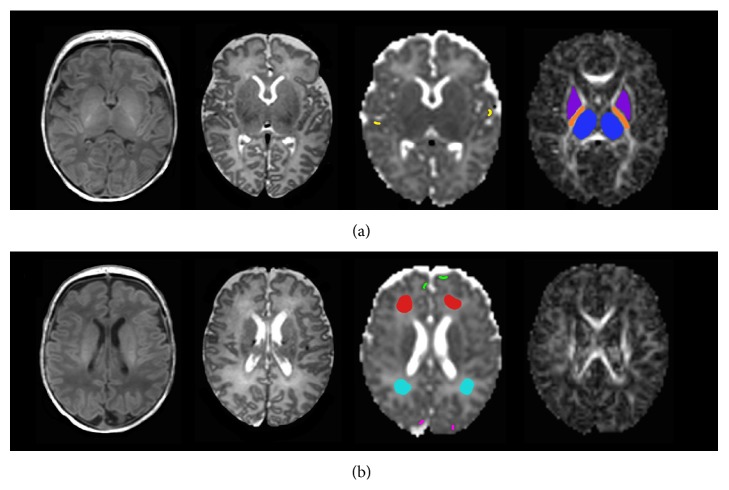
Regions of interest. Axial T1-weighted images, T2-weighted images, apparent diffusion coefficient (ADC) maps, and fractional anisotropy (FA) maps at two different levels of cerebrum showing the manually drawn regions of interest: (a) lower level and (b) higher level. ADC and FA values were measured in eight different regions of interest in the cerebrum: that is, in the thalamus, the posterior limb of the internal capsule and the lentiform nucleus, the white matter (within the frontal and posterior white matter), and the cortical grey matter (within the frontal, parietal, and occipital cortices). Regions of interest were always drawn by the same observer by looking at the same time at the ADC and FA maps and the corresponding T1- and T2-weighted imaging.
